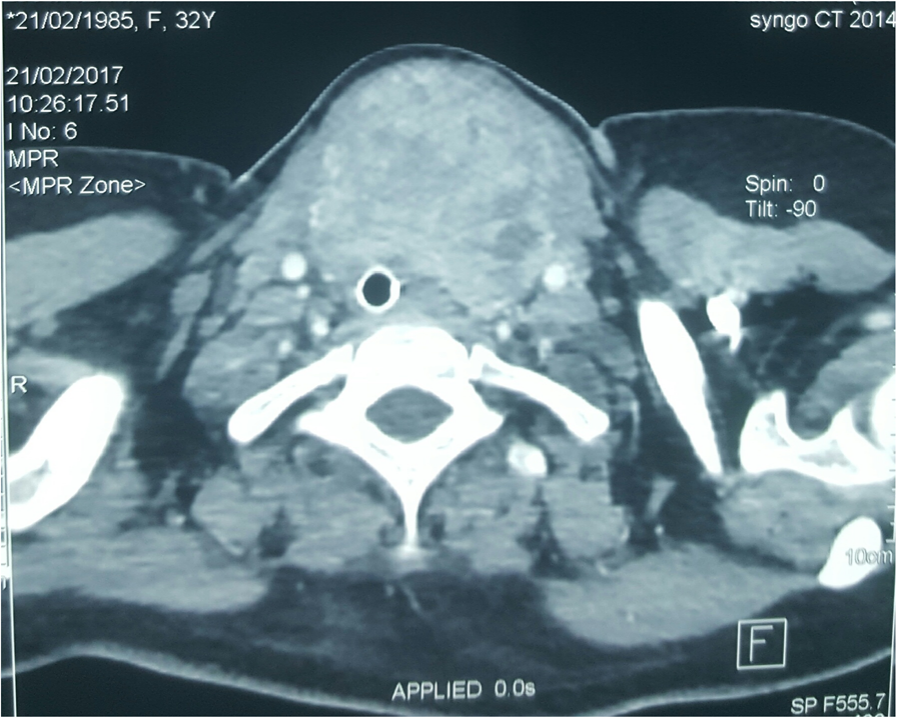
Computed tomography scan showing a huge goitre
